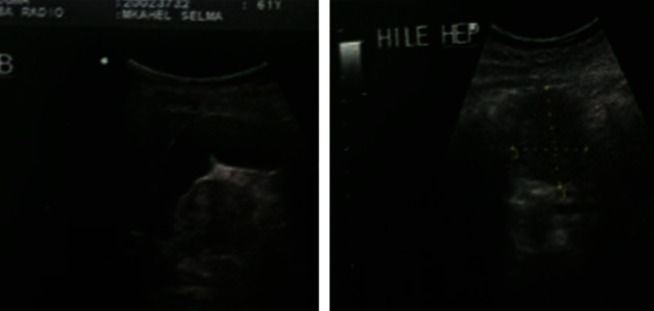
Abdominal ultrasound showing heterogeneous retroperitoneal nodular lesion, vascularized using color Doppler in presence of multiple partitions.
